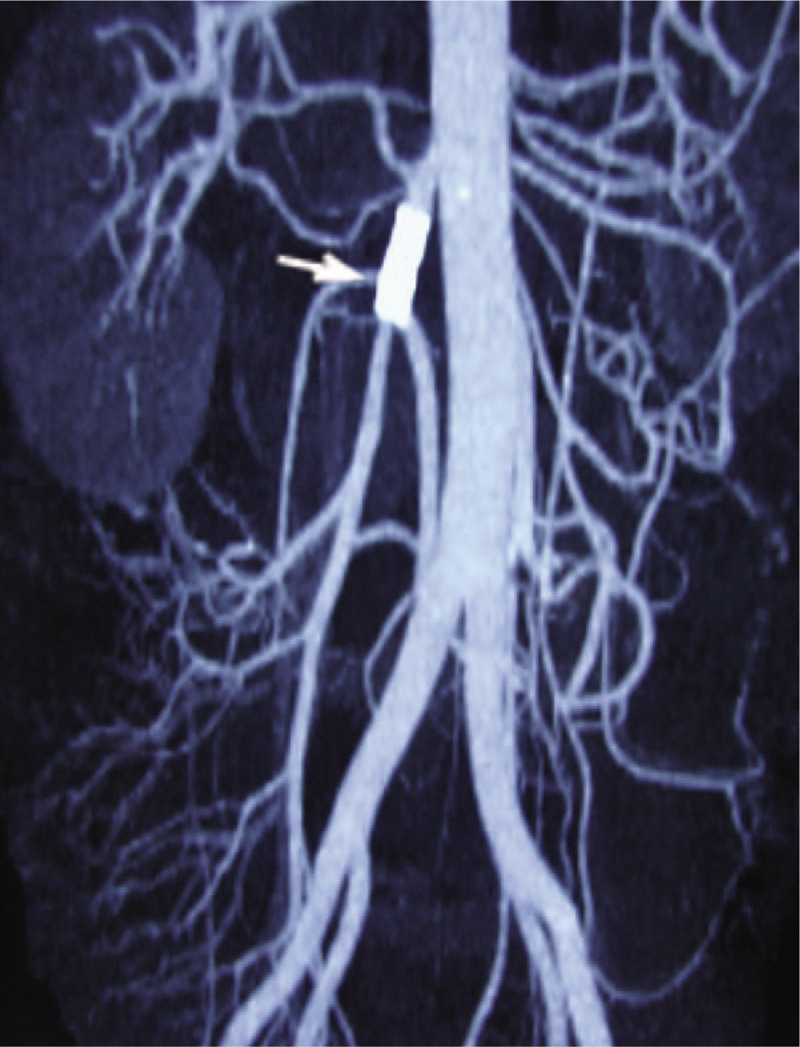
Six months later, follow-up computed tomography angiography (CTA) examination revealed stent patency without stenosis (white arrow).
